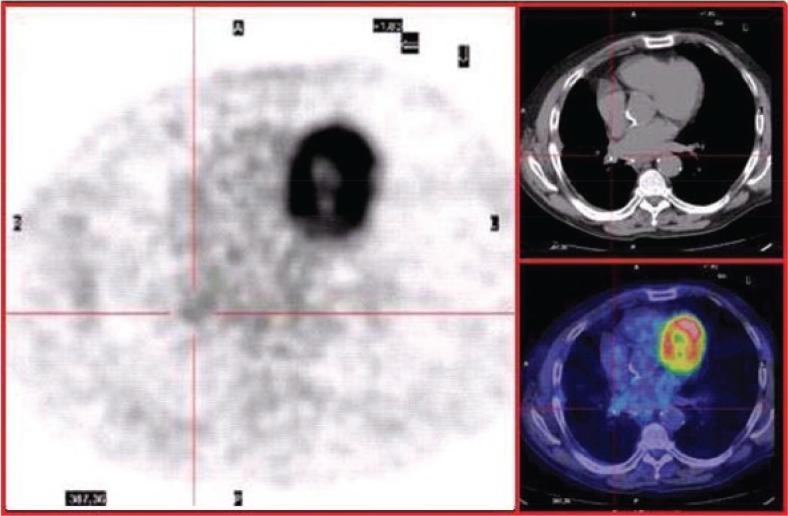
Post-surgical ^18^F-FDG PET/CT axial images. No pathological ^18^F-FDG uptake can be detected in the perihilar region of the right lung.
